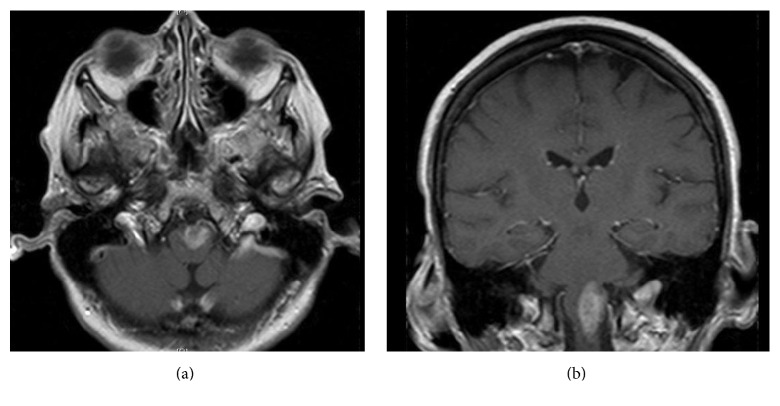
Brain MRI 2 weeks after the initial MRI. (a) Axial T1-weighted image with gadolinium, showing the enhancing left medullary lesion increased in size. (b) Coronal T1-weighted image with gadolinium showing the same medullary lesion increased in size.
